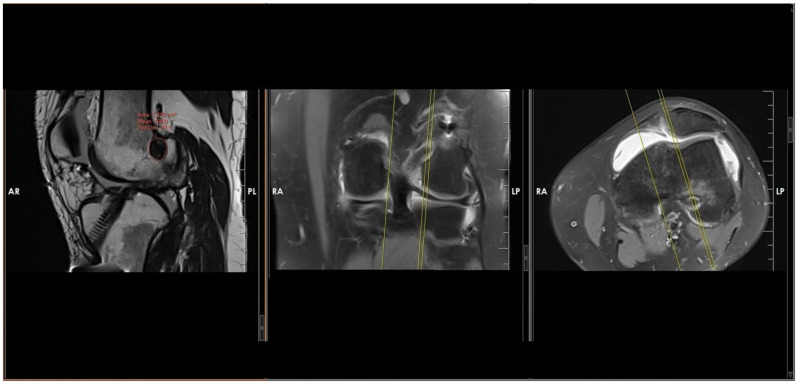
The femoral tunnel aperture on the joint surfaces of the femur was evaluated following anterior cruciate ligament reconstruction. Reference lines were used to identify the exact tunnel orientation, and the aperture area at the joint interface was measured using a freehand measurement tool (Uppercase letters and yellow lines are automatically generated by the MRI analysis software and represent measurement values and reference boundaries).
